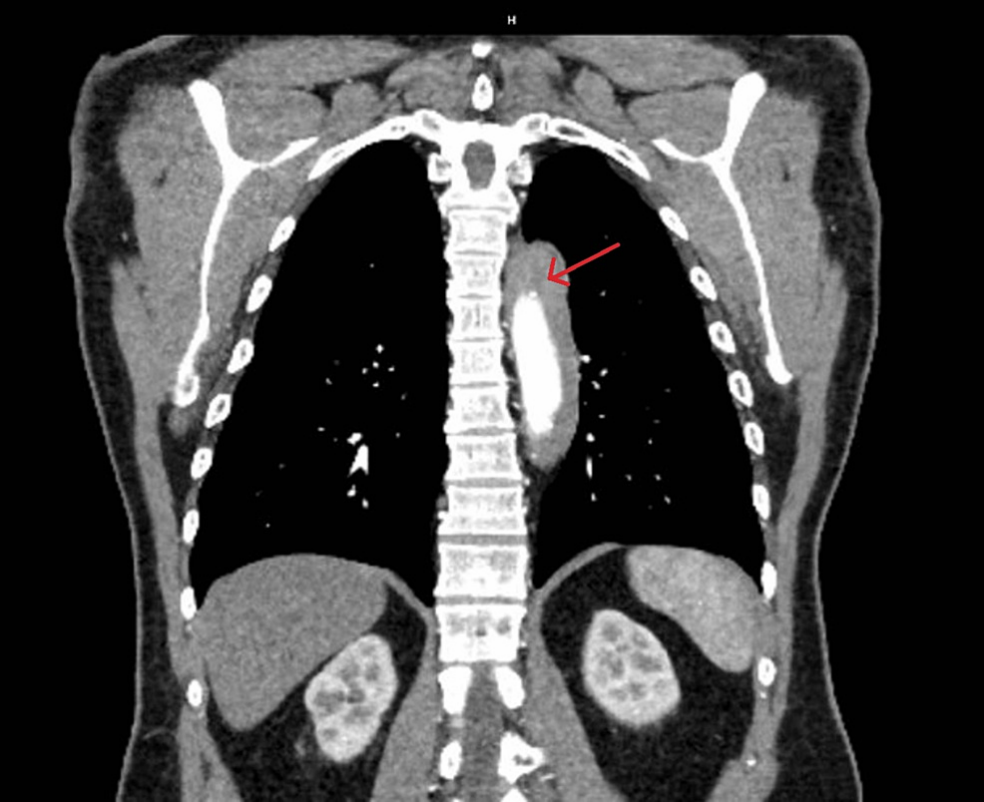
CT imaging showing a coronal view of the intramural hematoma surrounding the descending thoracic aorta (red arrow).
